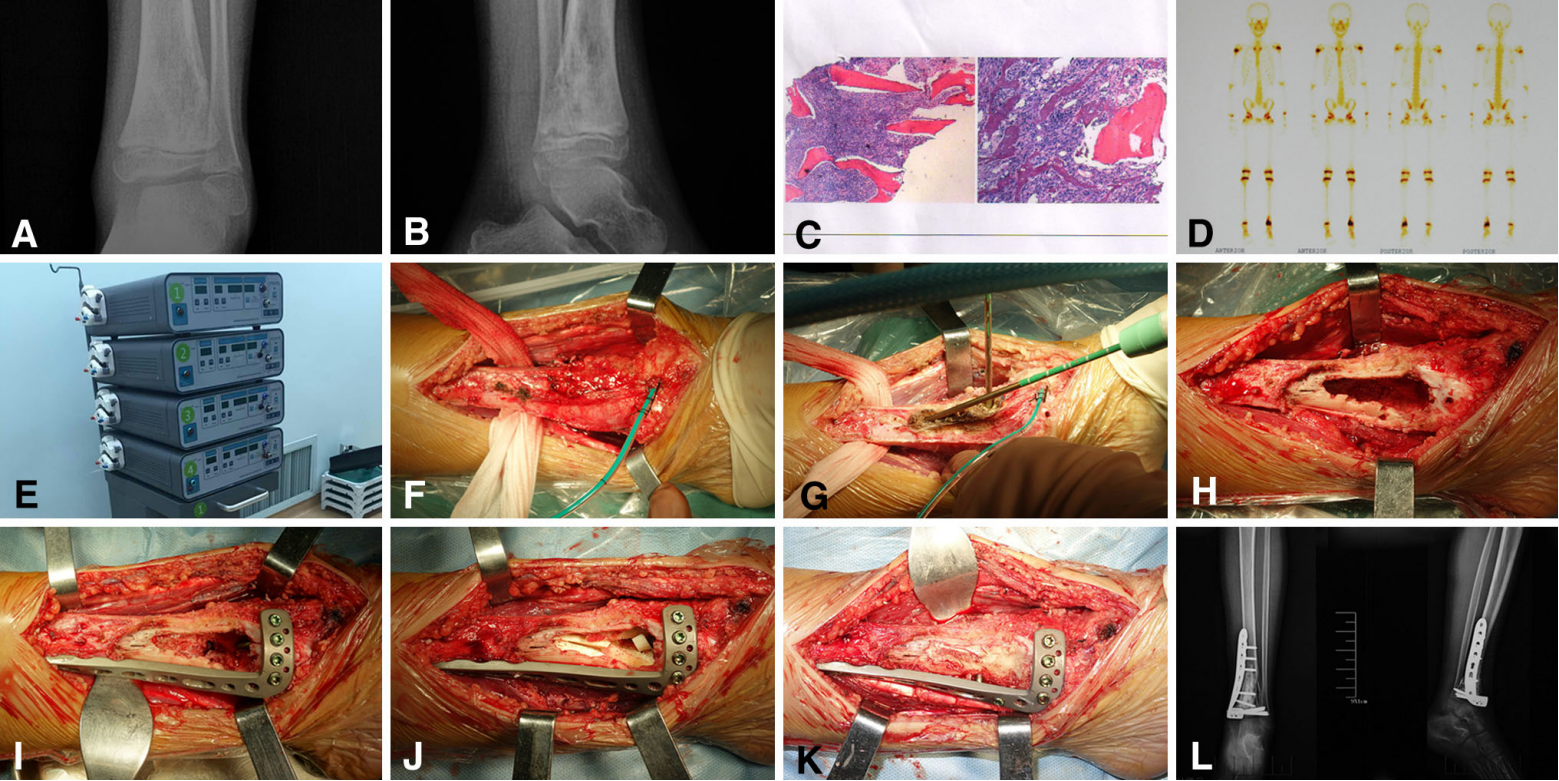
(A) AP and (B) lateral radiographs show an osteosarcoma of the distal tibia. (C) Pathologic examination of the tumor, and (D) bone scans are shown. (E) The photograph shows the microwave-induced hyperthermia machine. (F) Dissection is shown of the tumor-bearing bone from the surrounding normal tissues with a safe margin. (G) A wet gauze is placed between the tumor bone and surrounding normal tissues and electromagnetic energy is delivered to the tumor bone. (H) The dead or softened tumor is removed or curetted, (I) followed by prophylactic fixation. (J) The defect then is filled with allograft bone, (K) as shown in this image of the mixture of bone cement and allograft bone chips. (L) Postoperative AP and lateral radiographs are shown.
